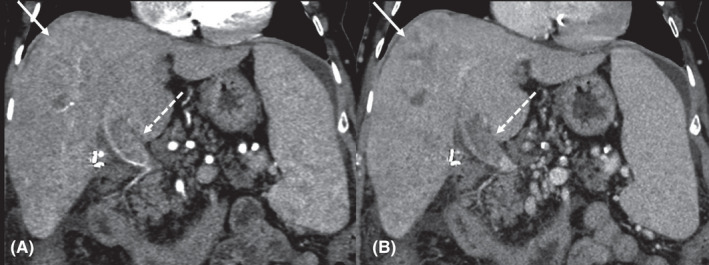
Image of a large HCC. Images from a contrast‐enhanced CT scan showing an HCC in a 66‐year‐old female cured of HCV using DAAs (Case 10). On CT in the coronal plane, an 11.4 cm ill‐defined HCC is present in the right lobe (arrows) on arterial (A) and portal venous phase (B). Enhancing portal vein tumor thrombus is also noted (dashed arrows). These findings were consistent with infiltrative HCC (LI‐RADS tumor‐in‐vein). Sequelae of portal hypertension, including splenomegaly and small volume abdominal ascites are also present; white dots are from cholecystectomy clips
